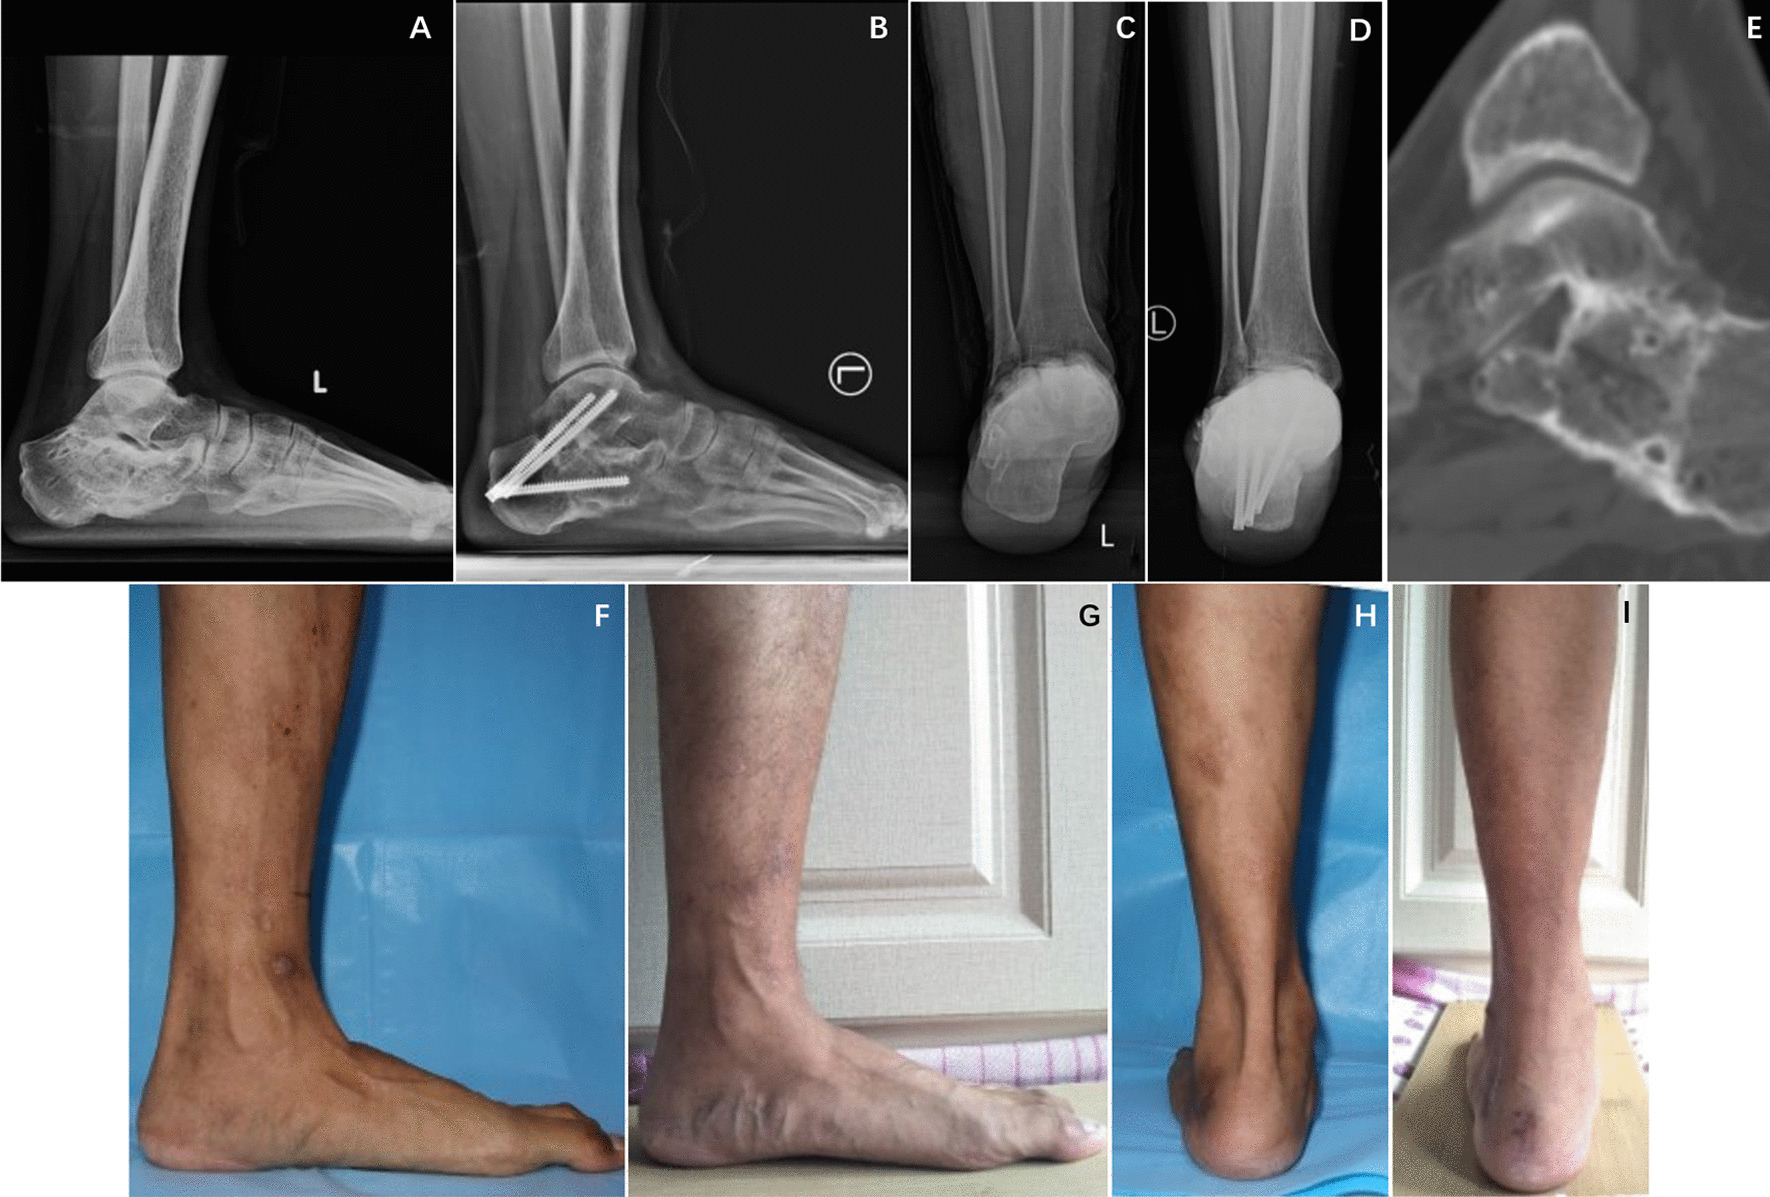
A 35-year-old male patient with left calcaneal malunion for 14 months was treated with Y-shaped calcaneal osteotomy and subtalar arthrodesis. A, B At the last follow-up, the calcaneal pitch angle, Böhler angle and talocalcaneal height were significantly improved compared with the values before the operation, and the osteotomy end and subtalar joint were osseously healed. C, D At the last follow-up, the calcaneal width and Hindfoot alignment angle were significantly improved compared with the values before the operation. E CT sagittal scan at the last follow-up showed bony union of the fusion site. F-I Comparison of external photographs of the patients before surgery and at the last follow-up
